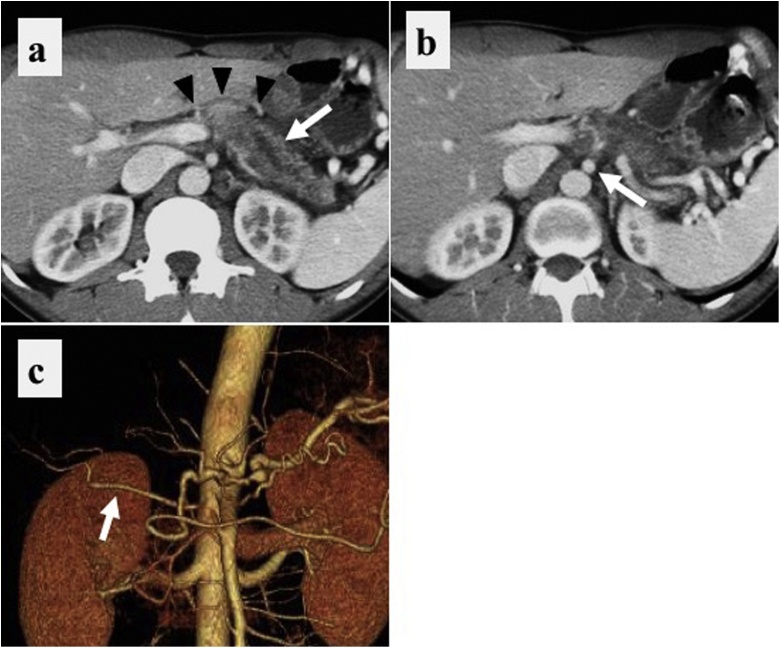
Preoperative computed tomography (Case #1) showed that. a: a hypodense lesion in the pancreatic body (black head) and dilation of the distal pancreatic duct (white head). b: the SMA free from the tumor (white arrow). c: the presence of the replaced RHA (white arrow).
